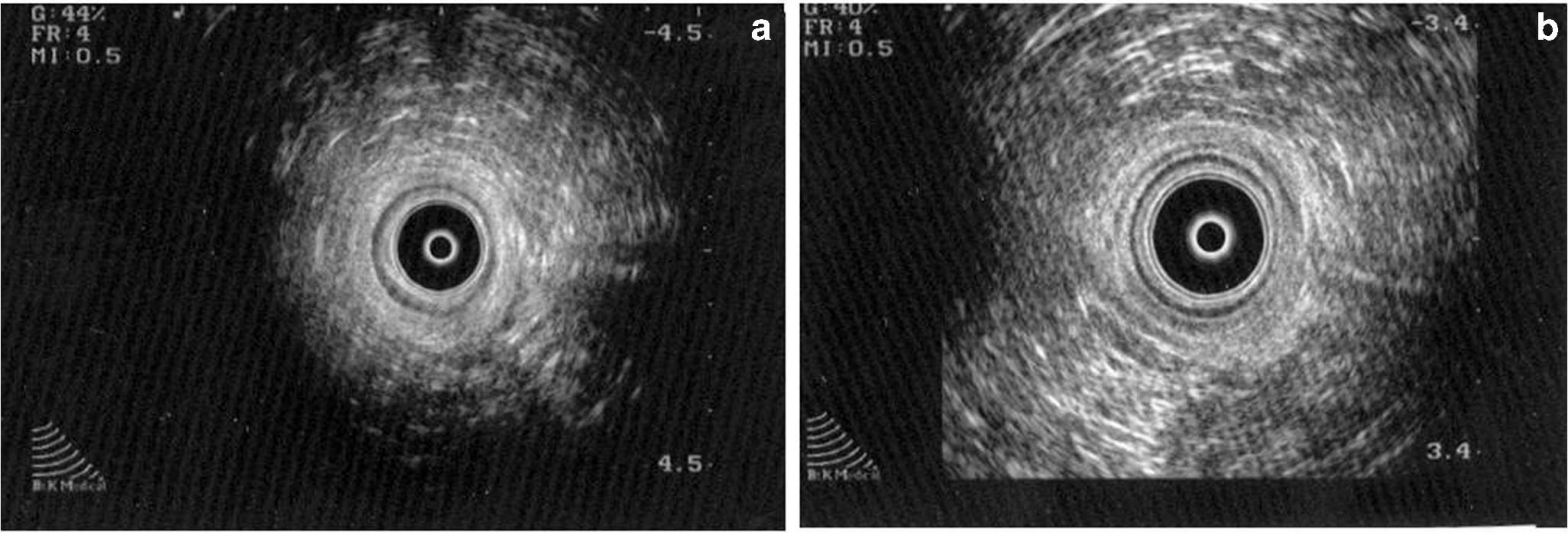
a Showing a normal sphincter complex on endoanal ultrasound. b Showing an anterior external sphincter defect following childbirth trauma. The internal sphincter is intact
